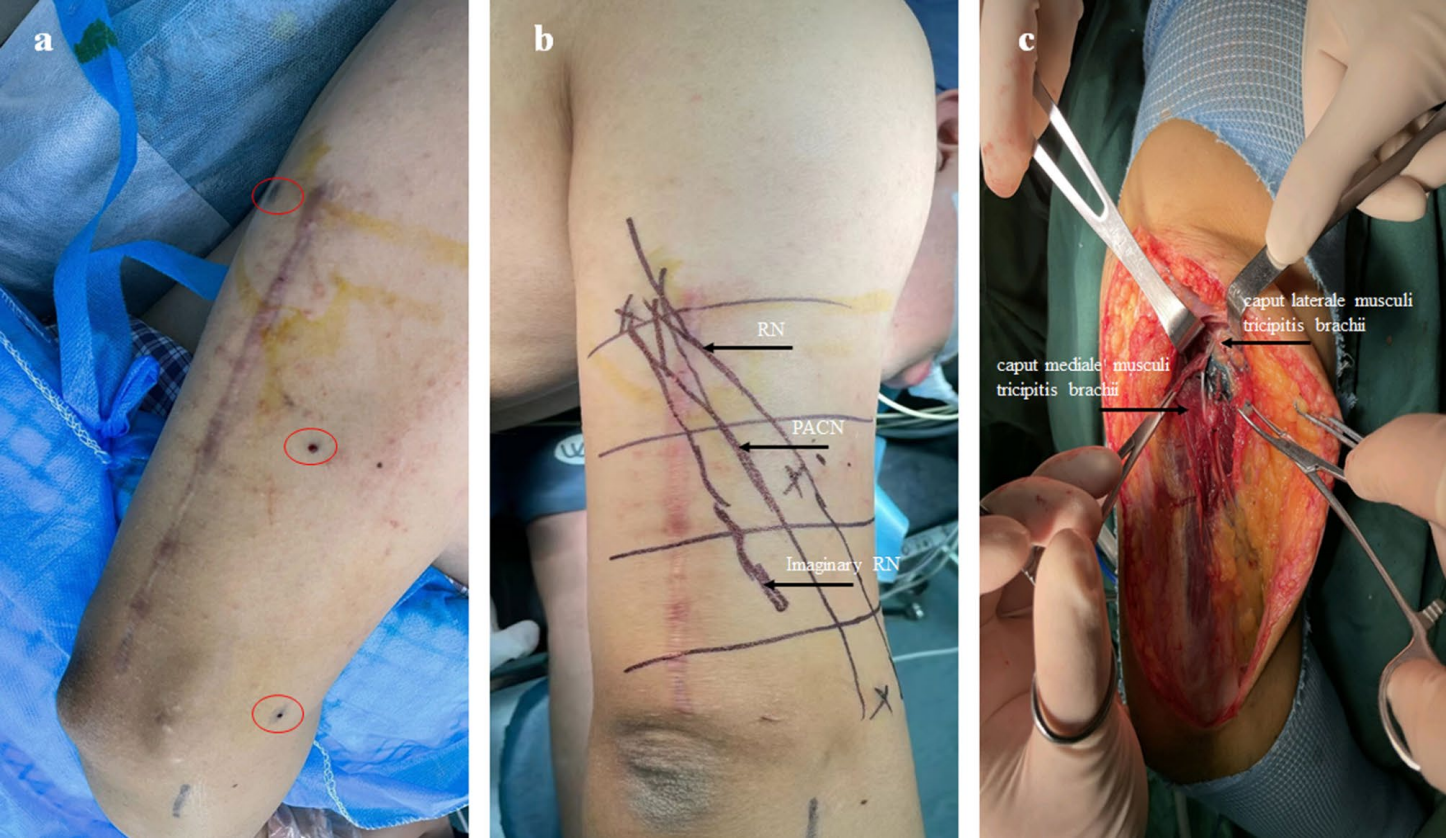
a Preoperative injection of methylthioninium chloride guided by ultrasound. b The body surface locations of the imaginary RN, PACN and RN were marked on the skin of the posterior upper arm; c Intraoperative image of the posterior upper arm
